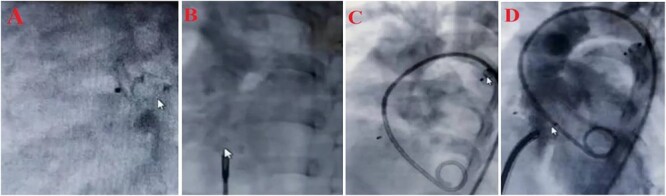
Treatment steps (A) close PDA by Amplatzer ADO II type AGA, size 5–6, (B) close ASD by Amplatzer Septal Oclotec size 15, (C) after closing PDA and ASD, D: close VSD by Amplatzer membranous, lifetec type symetric، size 12, (D) echocardiography four-chamber view after intervention.
